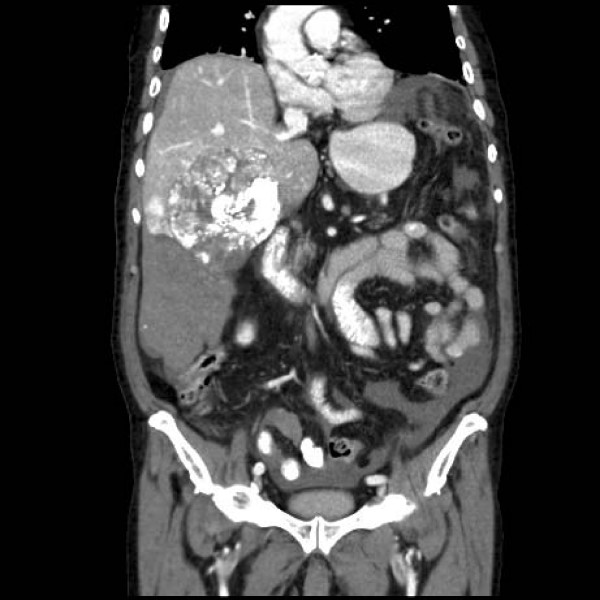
Computed tomographic scan of ruptured hepatocellular carcinoma with associated hemorrhage immediately post-TACE.
